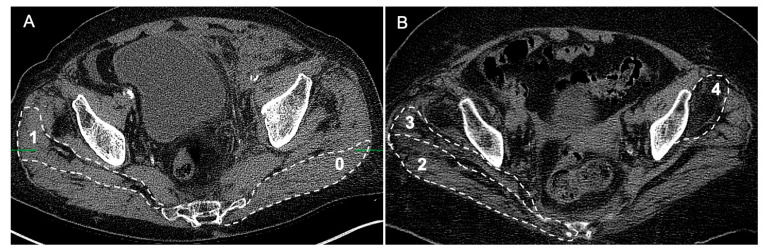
Examples of gMFA with different severities according to Goutallier grading scores based on CT pelvis images. (A) The area marked with 0 is an example of a score of 0 with no fat in the gluteus maximus, which was rare in the investigated population. The gluteus medius marked with 1 represented a score of 1 with intramuscular fatty steaks. (B) The area marked with 2 exemplified a score of 2 in gluteus maximus with mild atrophy, in which the proportion of fat infiltration was less than that of muscle. Nearby, an exaggerated fat infiltration could be found in the gluteus medius, marked by 3, where the proportion of fat and muscle were subjectively equal. The gluteus minimus, marked by 4, showed the most profound fat infiltration, where fat infiltration exceeded in quantity.
